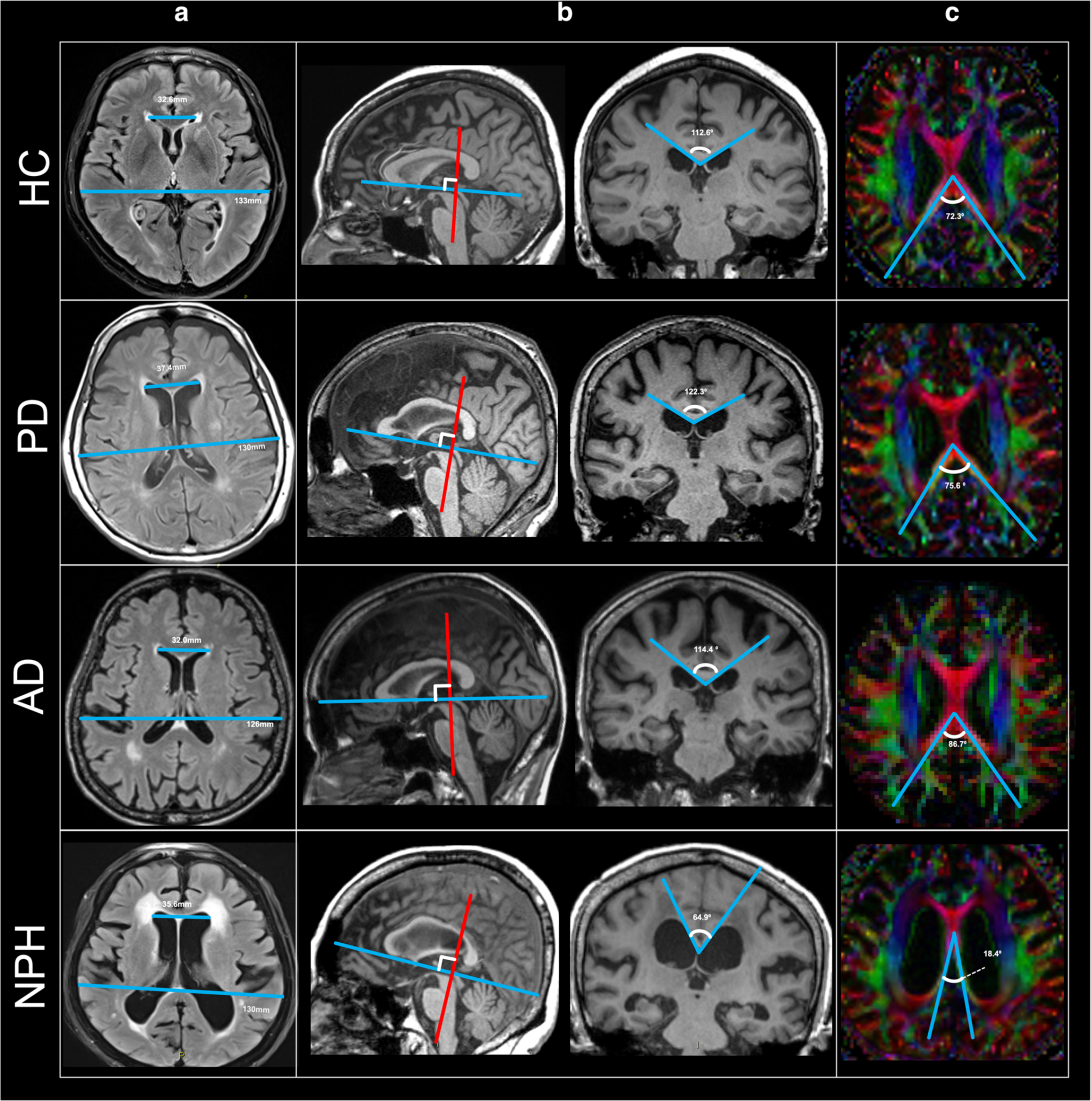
Evans’ index (EI), callosal angle (CA), and splenial angle (SA)measured on brain MRI in healthy controls (HC), Parkinson’s disease (PD), Alzheimer’s disease (AD), and normal pressure hydrocephalus (NPH) patients. a Axial FLAIR images demonstrating EI as a ratio of the maximal width of the frontal horns of the lateral ventricles (short blue line) against the maximal internal diameter of the cranium (long blue line) on the same section. b Left sagittal images depicting the coronal plane (red line) orthogonal to the anterior-posterior commissural (AC-PC) plane (blue line), and right coronal images demonstrating the CA subtended against the roof of the lateral ventricles. c Axial diffusion tensor imaging (DTI) color FA images containing the complete red-encoded callosal body depicting the SA subtended over the limbs of the forceps major and pivoted over the midline. Note the severely narrowed SA in an NPH patient arising from deformation of the red-encoded posterior callosal commissural fibers secondary to gross ventricular distension
